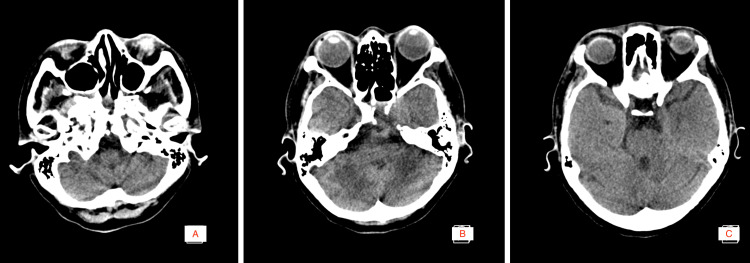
Pre-operative cranial CT scan A-C: (axial view) Bony anatomy relevant to preoperative planning denoting pneumatization of the petrous bone.
